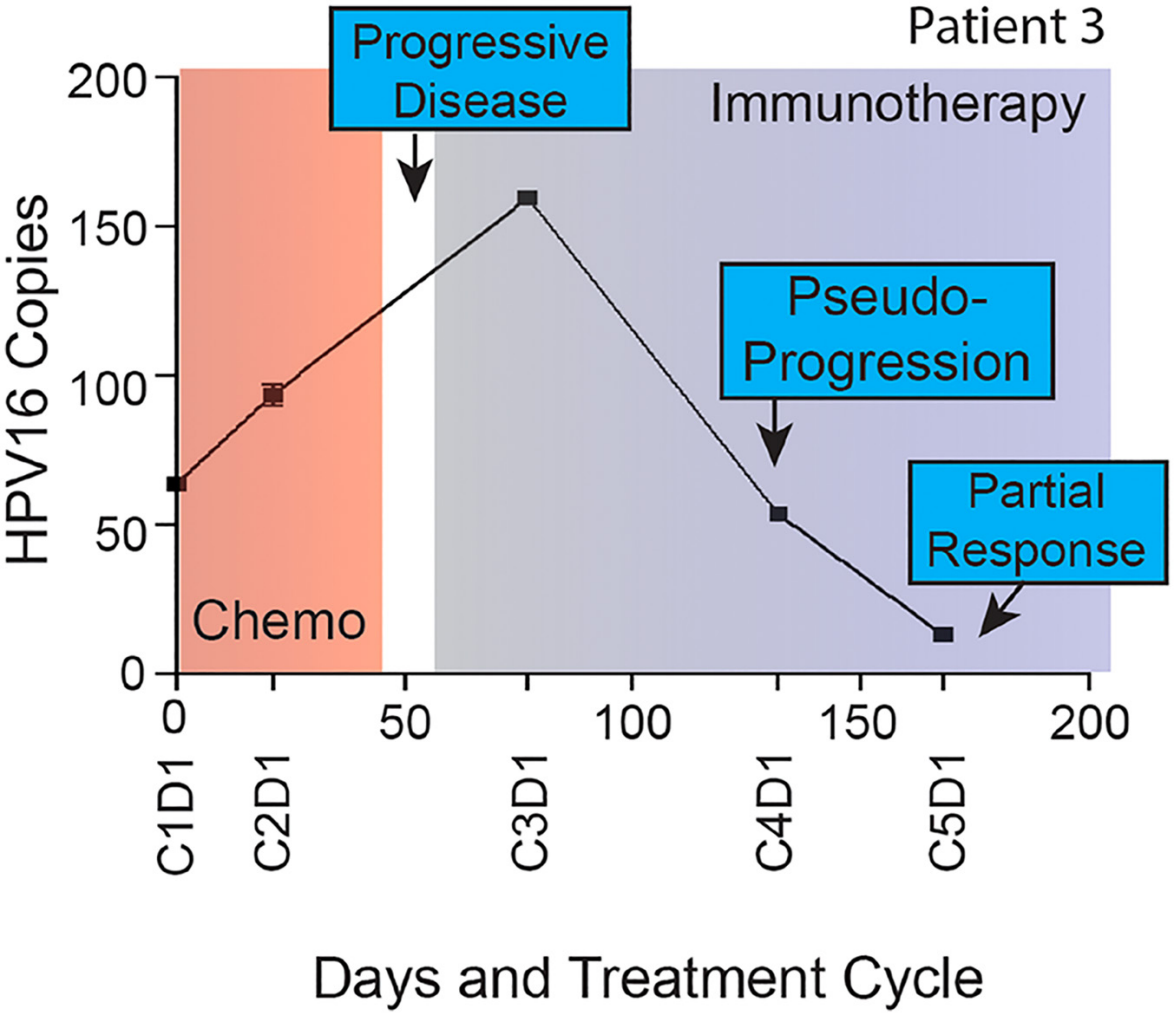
Longitudinal ctHPV-DNA levels in a patient with HPV-16+ malignancy treated with unspecified immunotherapy. Plasma HPV16 ctDNA (copies per l uL of plasma) measured over time in a patient with p16+ recurrent/metastatic HPV+ OPSCC. On the x-axis are days since study enrollment as well as cycle and day (CxDx) of treatment in a patient with radiographic pseudo-progression while treated with immunotherapy. Figure from Haring CT, Oncotarget 2021 [23]. Copyright © Haring et al.
